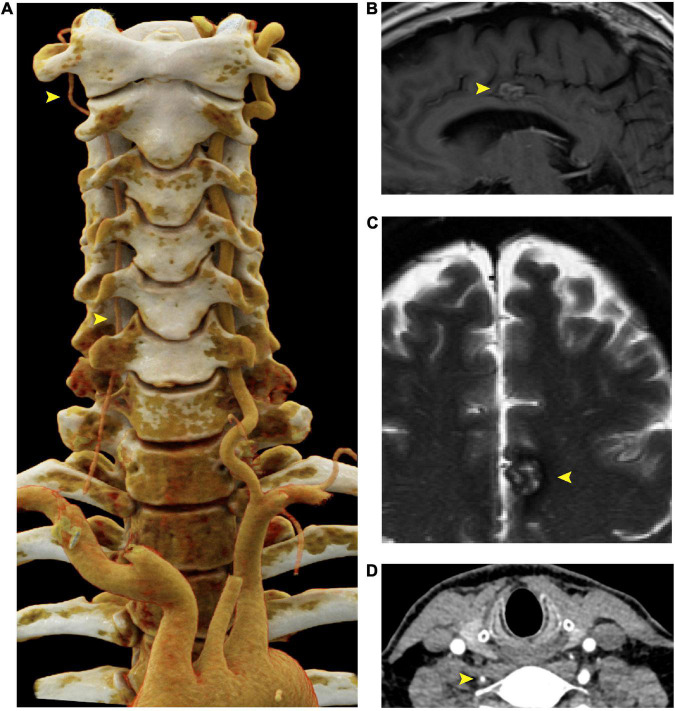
Right vertebral artery atresia and cavernoma in a 55 year old male harboring a *SMAD3* V244F variant. (A) Cinematic 3D reformatted images of the atretic < 2mm right vertebral artery. The origin of the right vertebral artery was sufficiently narrow in caliber that volume averaging resulted in below-threshold density required for visualization in the reformat. For best visualization of the vertebral arteries, the carotid arteries were digitally removed using *syngo*.via (Siemens, Erlangen). The dominant left vertebral artery measures 5 mm in diameter throughout most of its length, which is the upper limit of normal. (B) Sagittal T1-weighted FLAIR MRI of the brain at the midline shows a cavernous malformation in the left mid cingulate gyrus, just superior to the body of the corpus callosum. (C) Axial T2-weighted MRI with fat saturation demonstrates characteristic signal intensity of cavernous malformation with central hyperintensity, peripheral hypointensity, and susceptibility artifact. (D) Axial CTA demonstrating atretic < 2mm right vertebral artery. FLAIR is fluid-attenuated inversion recovery, MRI is magnetic resonance imaging.
